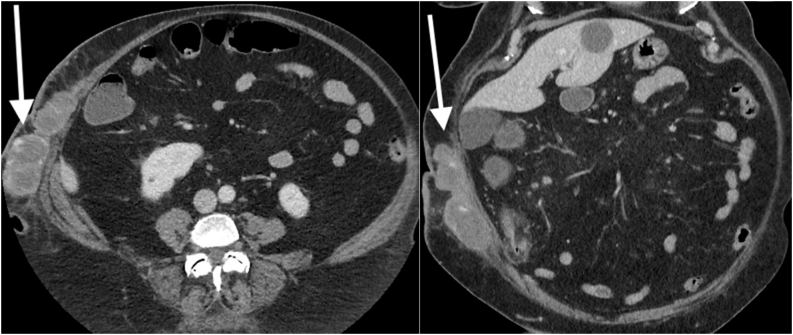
LEFT: Axial contrast-enhanced CT image shows the recurrence of a mass in the same area but is more extensive, lobulated and nodular in appearance. RIGHT: Coronal CT image of the same lesion.
